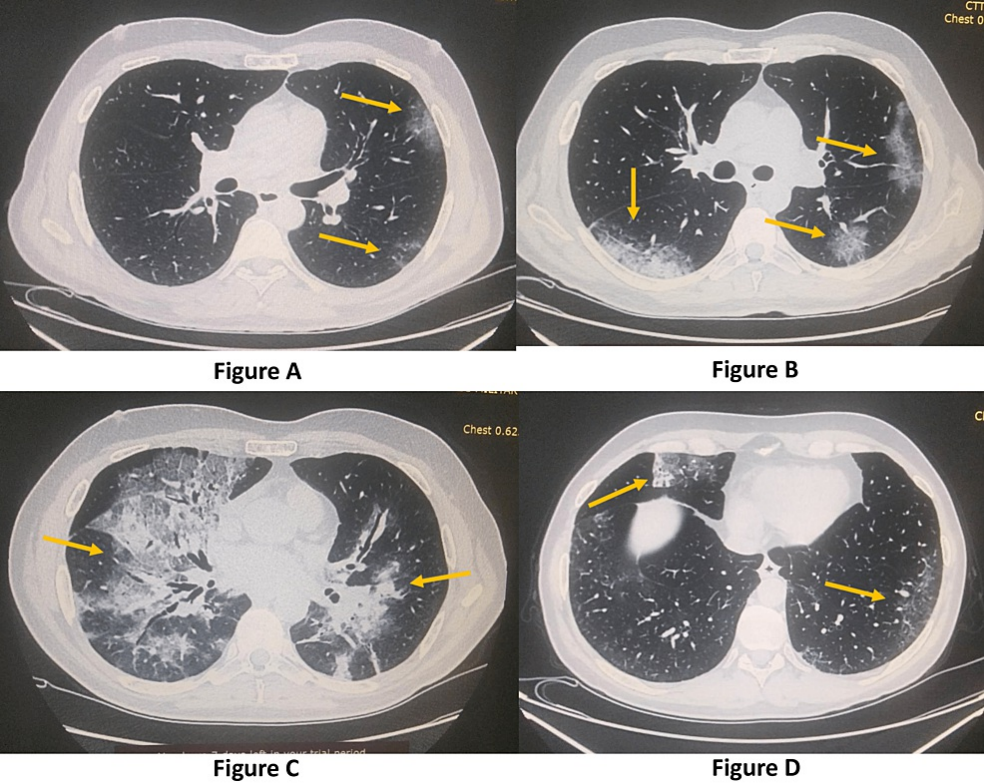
Chest computed tomography (CT) at various points of the disease. Figure 1A: Initial patient presentation at the ED and admission.
